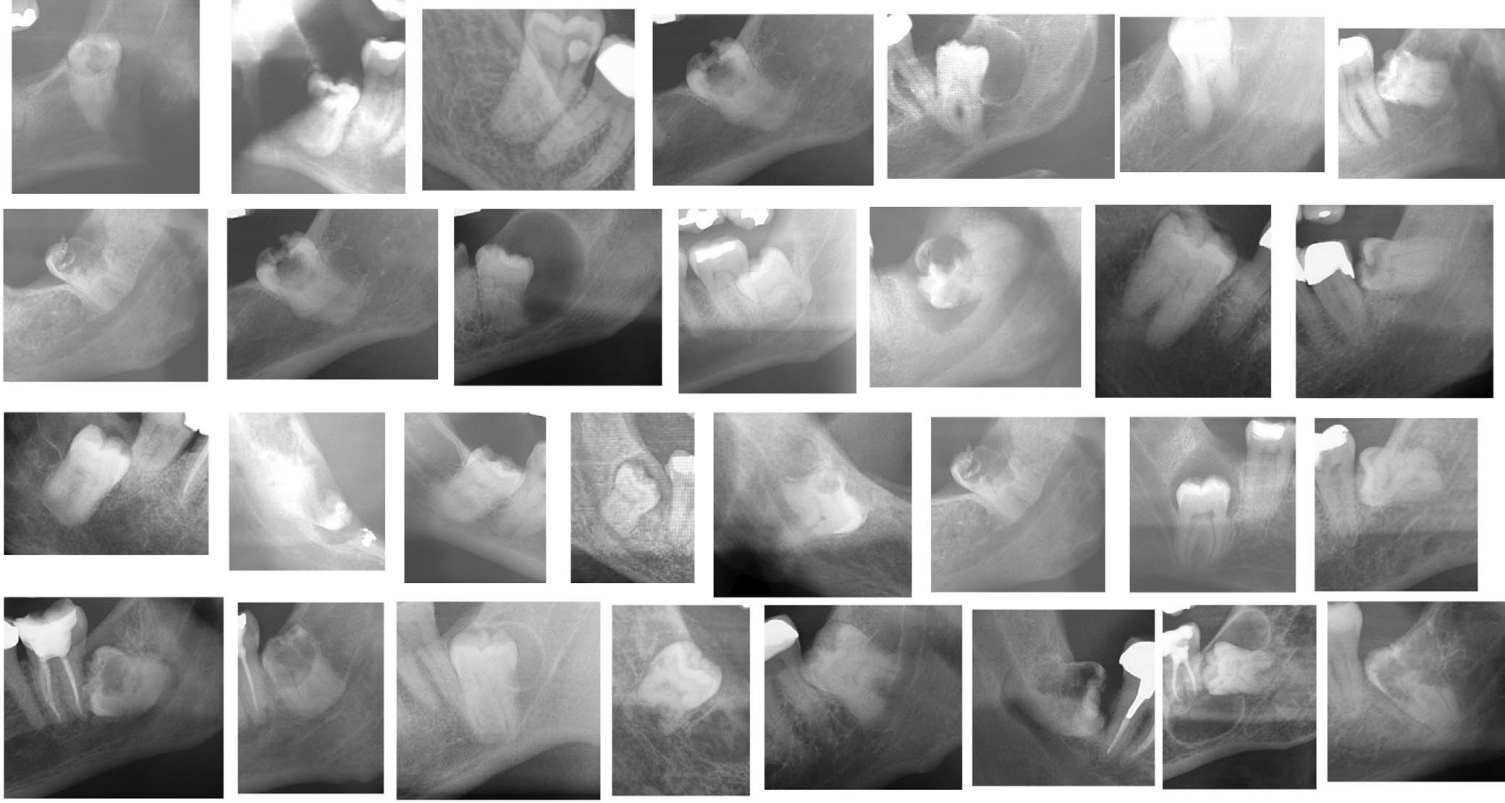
The preoperative section of the panoramic radiographs of the included patients.
